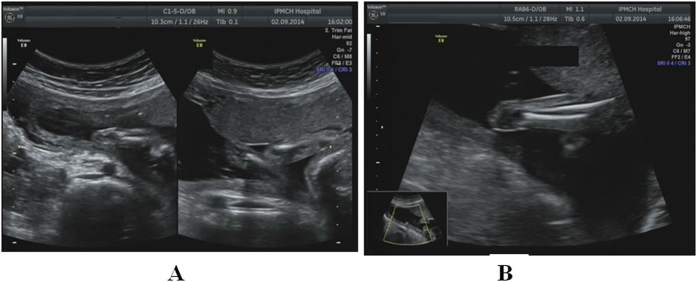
Absence of both hands at a gestational age of 22 weeks. (A) The metacarpal bone plane and fingers were invisible at the termini of both forearms, and the wrists were blind-ended. (B) Two-dimensional sonogram showing the ulna and radius in the right forearm, but the metacarpal bone plane and fingers cannot visible at any time.
